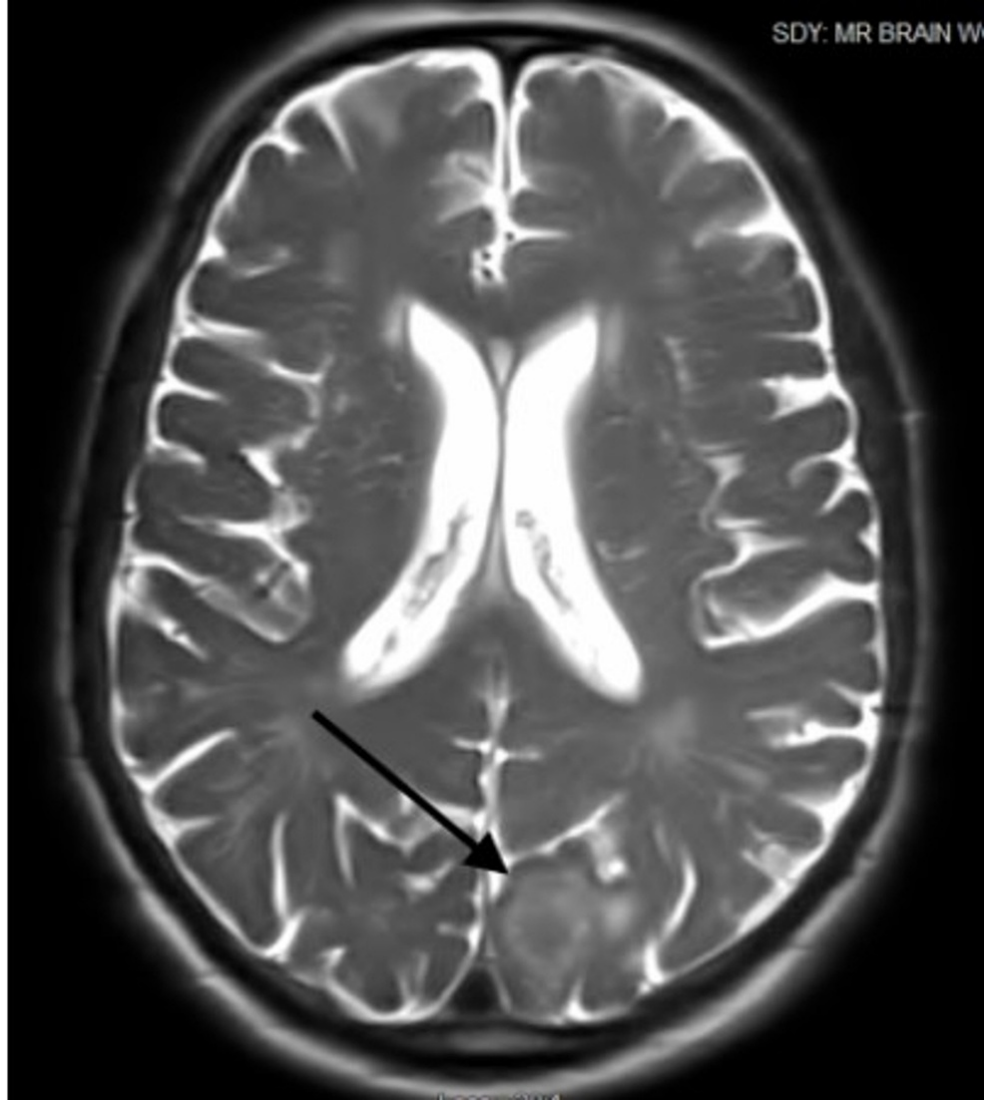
A left occipital lobe-enhancing solid mass measuring 1.5 × 1.7 cm with mild-to-moderate vasogenic edema is seen on axial T2 MRI of the brain. MRI: magnetic resonance imaging
